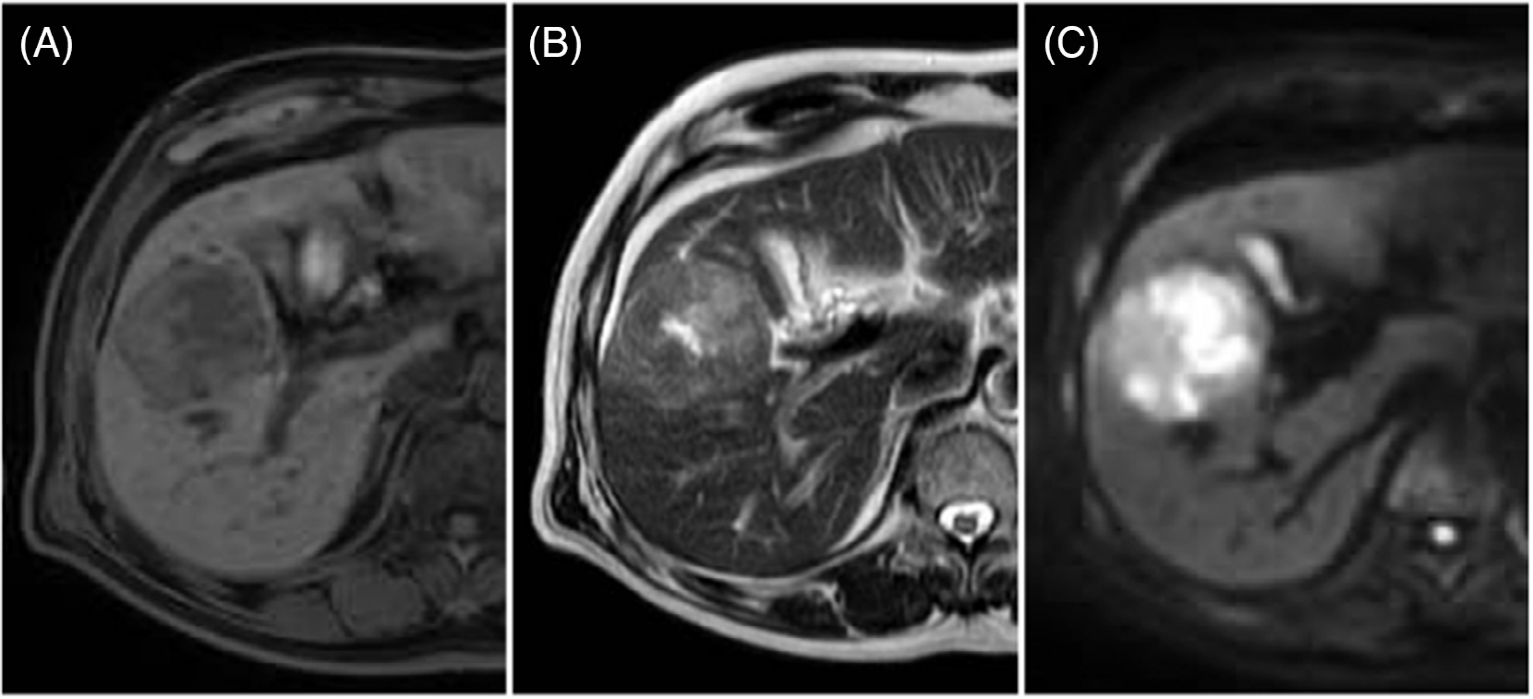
Results of magnetic resonance imaging: Tumor showing low and high signal intensity on T1‐weighted images (A) and T2‐weighted images (B), respectively. Diffusion‐weighted images revealing high signal intensity in the central part of the tumor, corresponding to the low‐density area detected through enhanced computed tomography (C).
